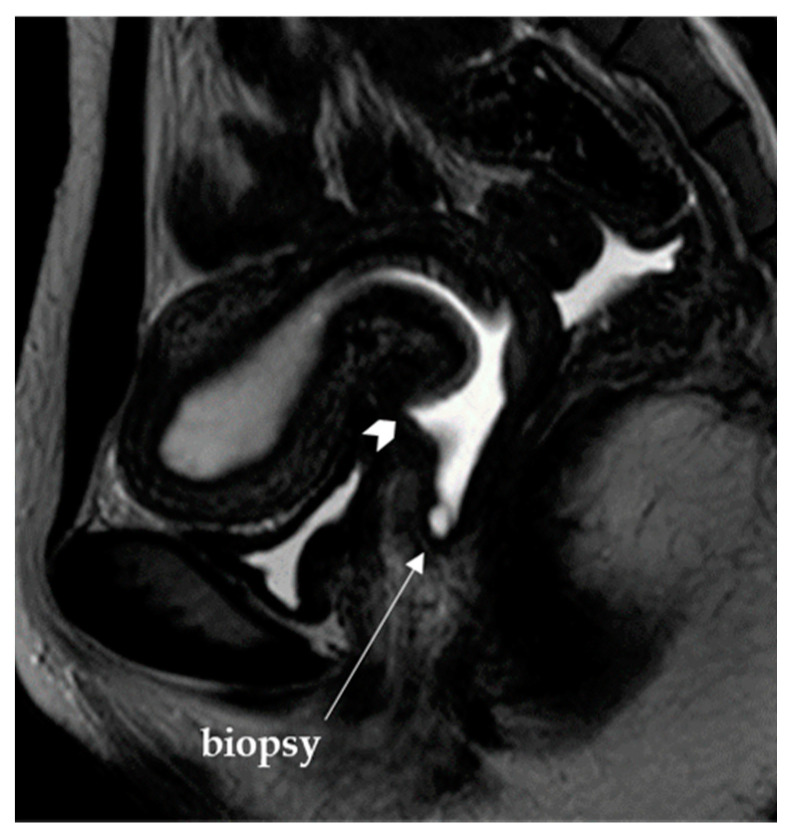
The sagittal T2-weighted magnetic resonance image shows a definite contour of the cervix (arrowhead), suggesting vaginal agenesis with fluid collection in Patient 15 (Table 2). However, the intraoperative biopsy, which was performed at the caudal leading edge (arrow), revealed the presence of cervical gland and stroma material.
